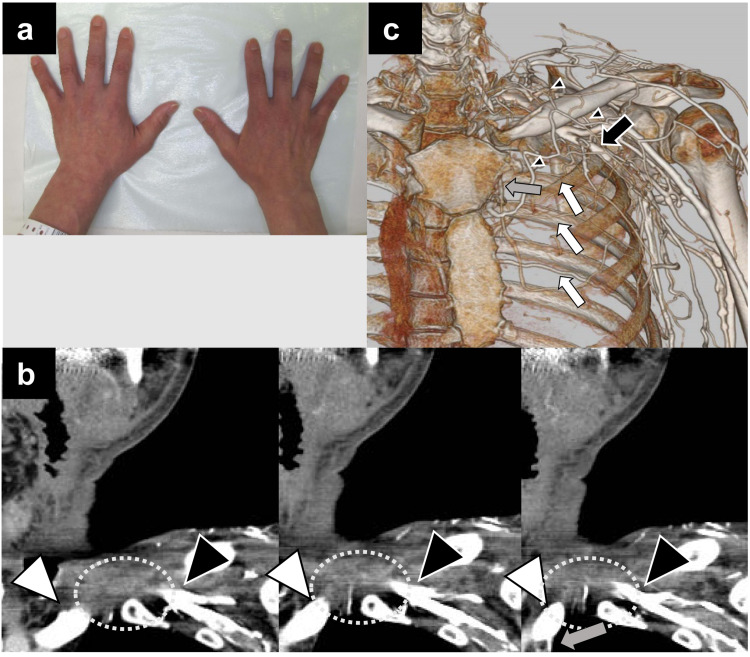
Hemodynamics after resection of brachiocephalic and subclavian veins. (a) Postoperative photograph of the hands showing there is no edema. (b) Contrast-enhanced coronal CT image showing disruption of the proximal subclavian vein (black arrow-heads) and distal brachiocephalic vein (white arrow-heads). Gray arrow indicates the inflow from the internal thoracic vein. Dashed circles indicate the tumor resection area. (c) 3-D volume rendered image with contrast medium. White arrows indicate the intercostal vein and gray arrow indicates the internal thoracic vein. Note the small blood vessels branching from the axillary vein (black arrow) to the dorsal, lower neck, and precordial regions (small black arrow-heads).
